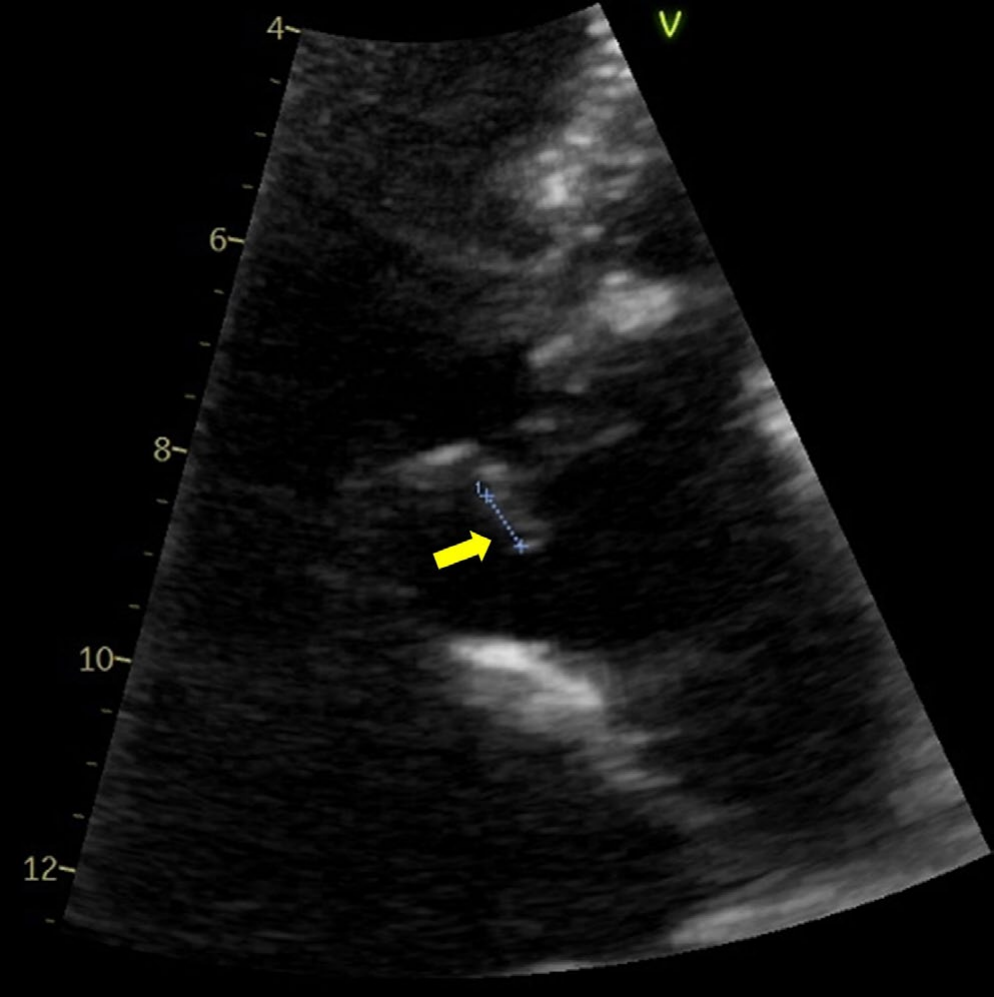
Transthoracic echocardiography showing a 5.6‐mm vegetation attached to the aortic valve (arrow).
